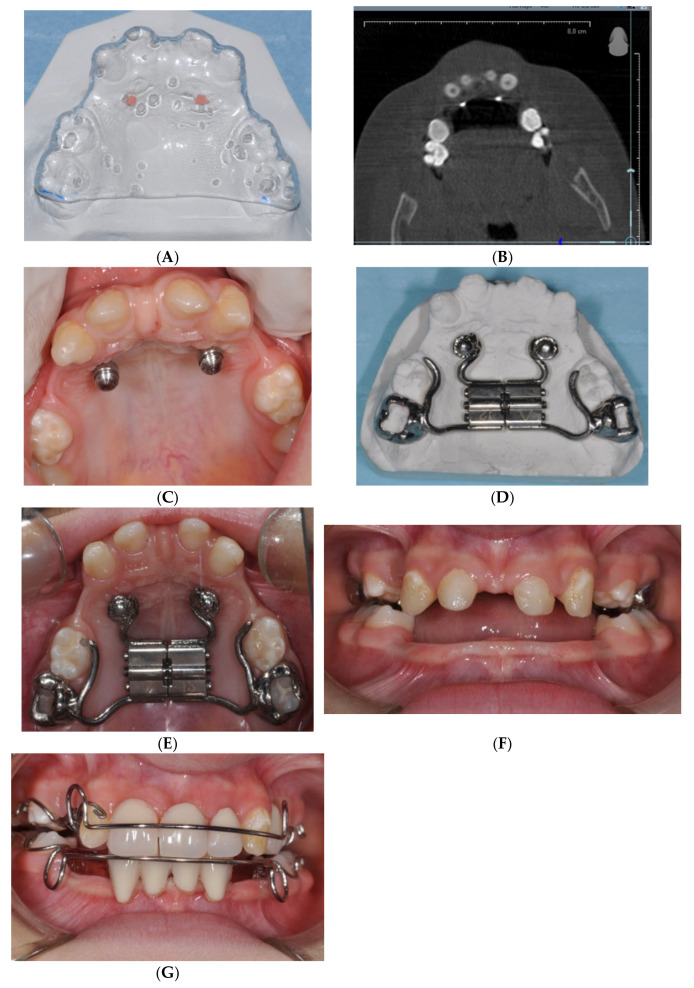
(A) Customized surgical guides for hypihydrotic ED patient. (B) CBCT scan performed for evaluation of bone quality and quantity for hypihydrotic ED patient. (C) Miniscrew placement in hypihydrotic ED patient. (D) Customized hybrid MSE for hypihydrotic ED patient. (E) MSE application for hypihydrotic ED patient. (F) MSE application for hypihydrotic ED patient. (G) Design and application of Schwarz appliances incorporated with dental elements in ED patient.
